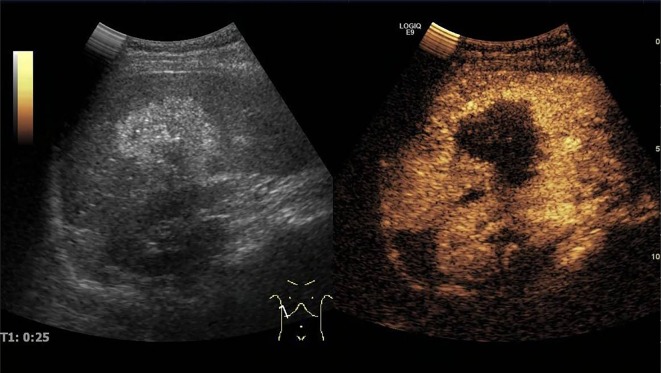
Contrast-enhanced Ultrasonography (CEUS) using SonoVue*^®^*in a case of hepatic alveolar echinococcosis.* CEUS showed a hepatic mass with irregular rim enhancement in the peripheral areas and no enhancement within the central areas at the hepatic artery phase (25 s).
